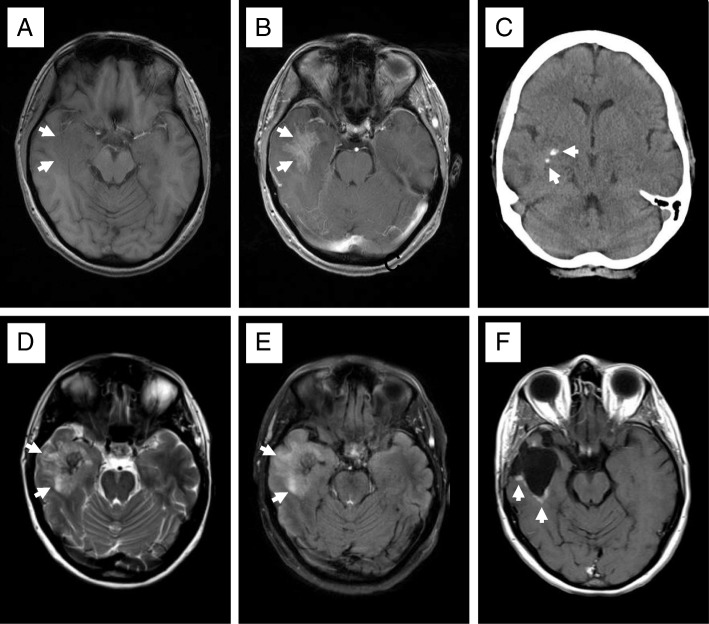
Neuroradiologic imaging pattern of primary brain amyloidoma. The T1-weighted MRI sequence shows a slightly hypointense right temporal mass (arrows in a), an ill-defined, moderate gadolinium enhancement (arrows in b), both compatible with malignant glioma. The CT displays small focal calcifications (arrows in c). The corresponding T2- and FLAIR MRI sequences displayed a hyperintense signal extending beyond the margins of the contrast enhancement indicating peritumoral edema or an infiltrating part of the mass (arrows in d and e). Almost 2 years following surgical removal of the PBA, the T1-weighted MRI sequence shows contrast enhancement at the resection margins (arrows in f) compatible with residual amyloidoma that remained unchanged during the 8-year follow-up
